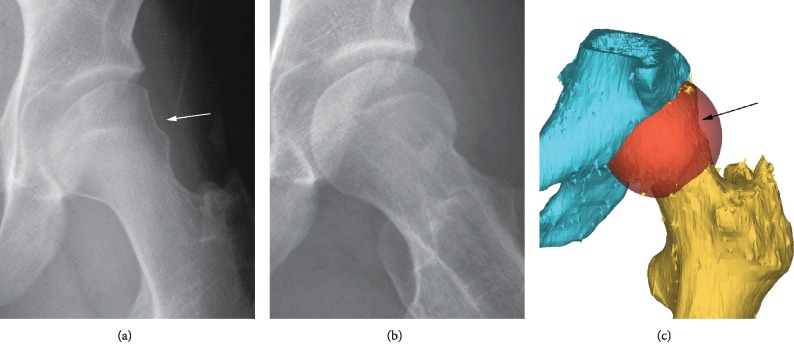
(a) Anteroposterior plain radiograph of the left hip demonstrating a femoral head deformity laterally (white arrow) with no signs of osteoarthritis. (b) Lateral plain radiograph demonstrating normal morphology of the femoral head with no signs of osteoarthritis. (c) Three-dimensional reconstruction of the pelvis (blue) and proximal femur (gold) with superimposed spherical virtual femoral head (orange) demonstrating the area of missing bone on the lateral femoral head (black arrow).
